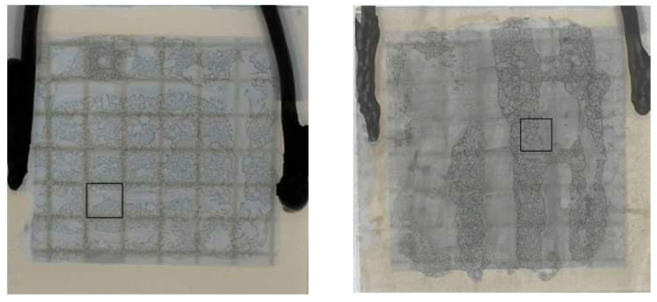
X radiography of the die attach for the two samples; presence of important voids for the etch patch one (right).
